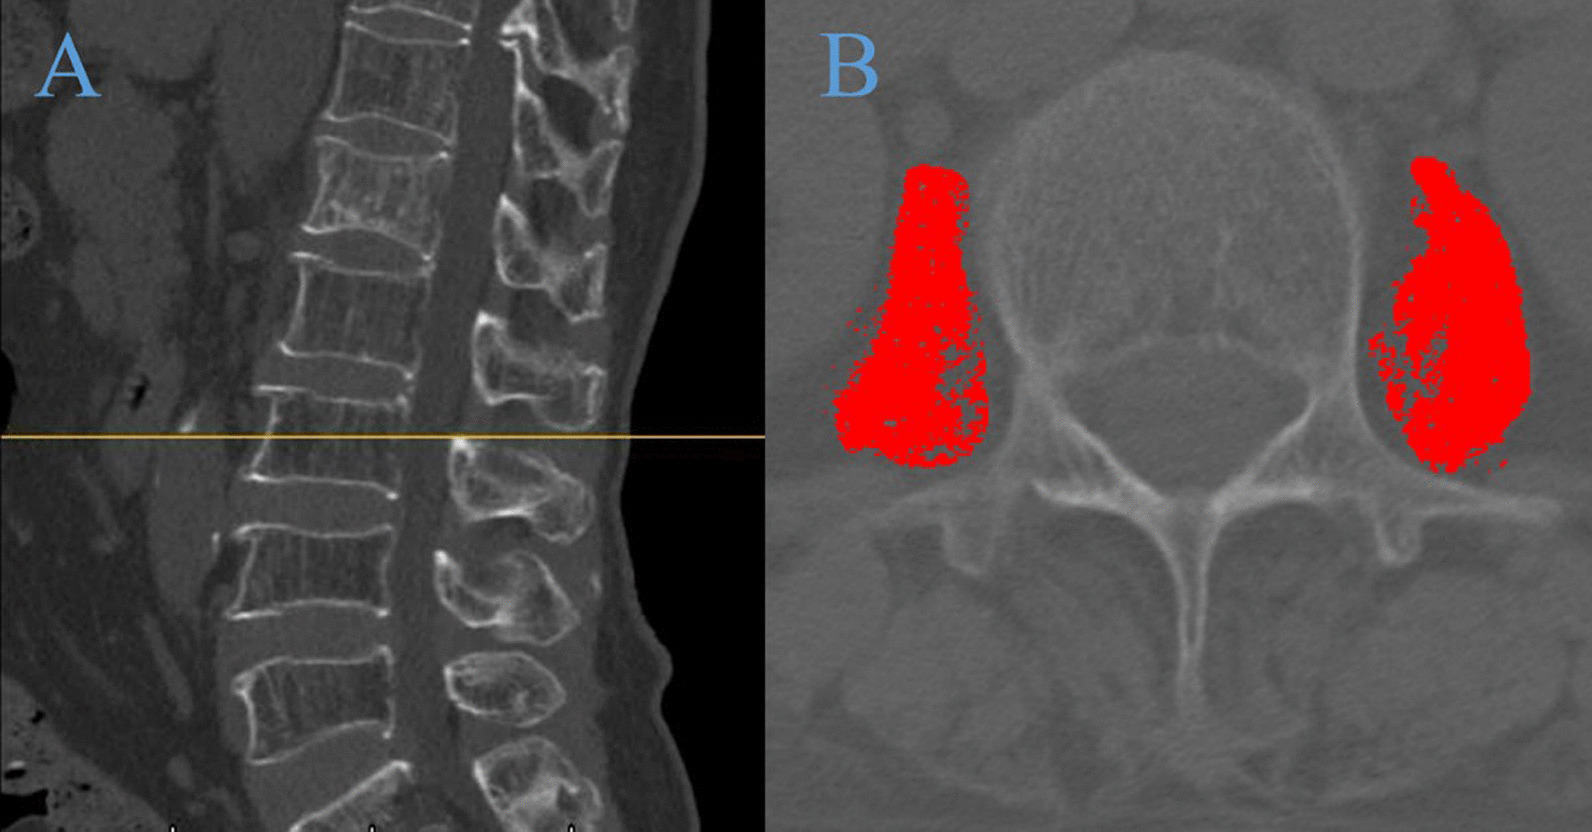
The TPA at level L3 was measured on CT by tracing the bilateral psoas major muscle outline. A sagittal views of Lumbar CT (L3, yellow line); B cross-sectional views of Lumbar CT (TPA, red fill)
